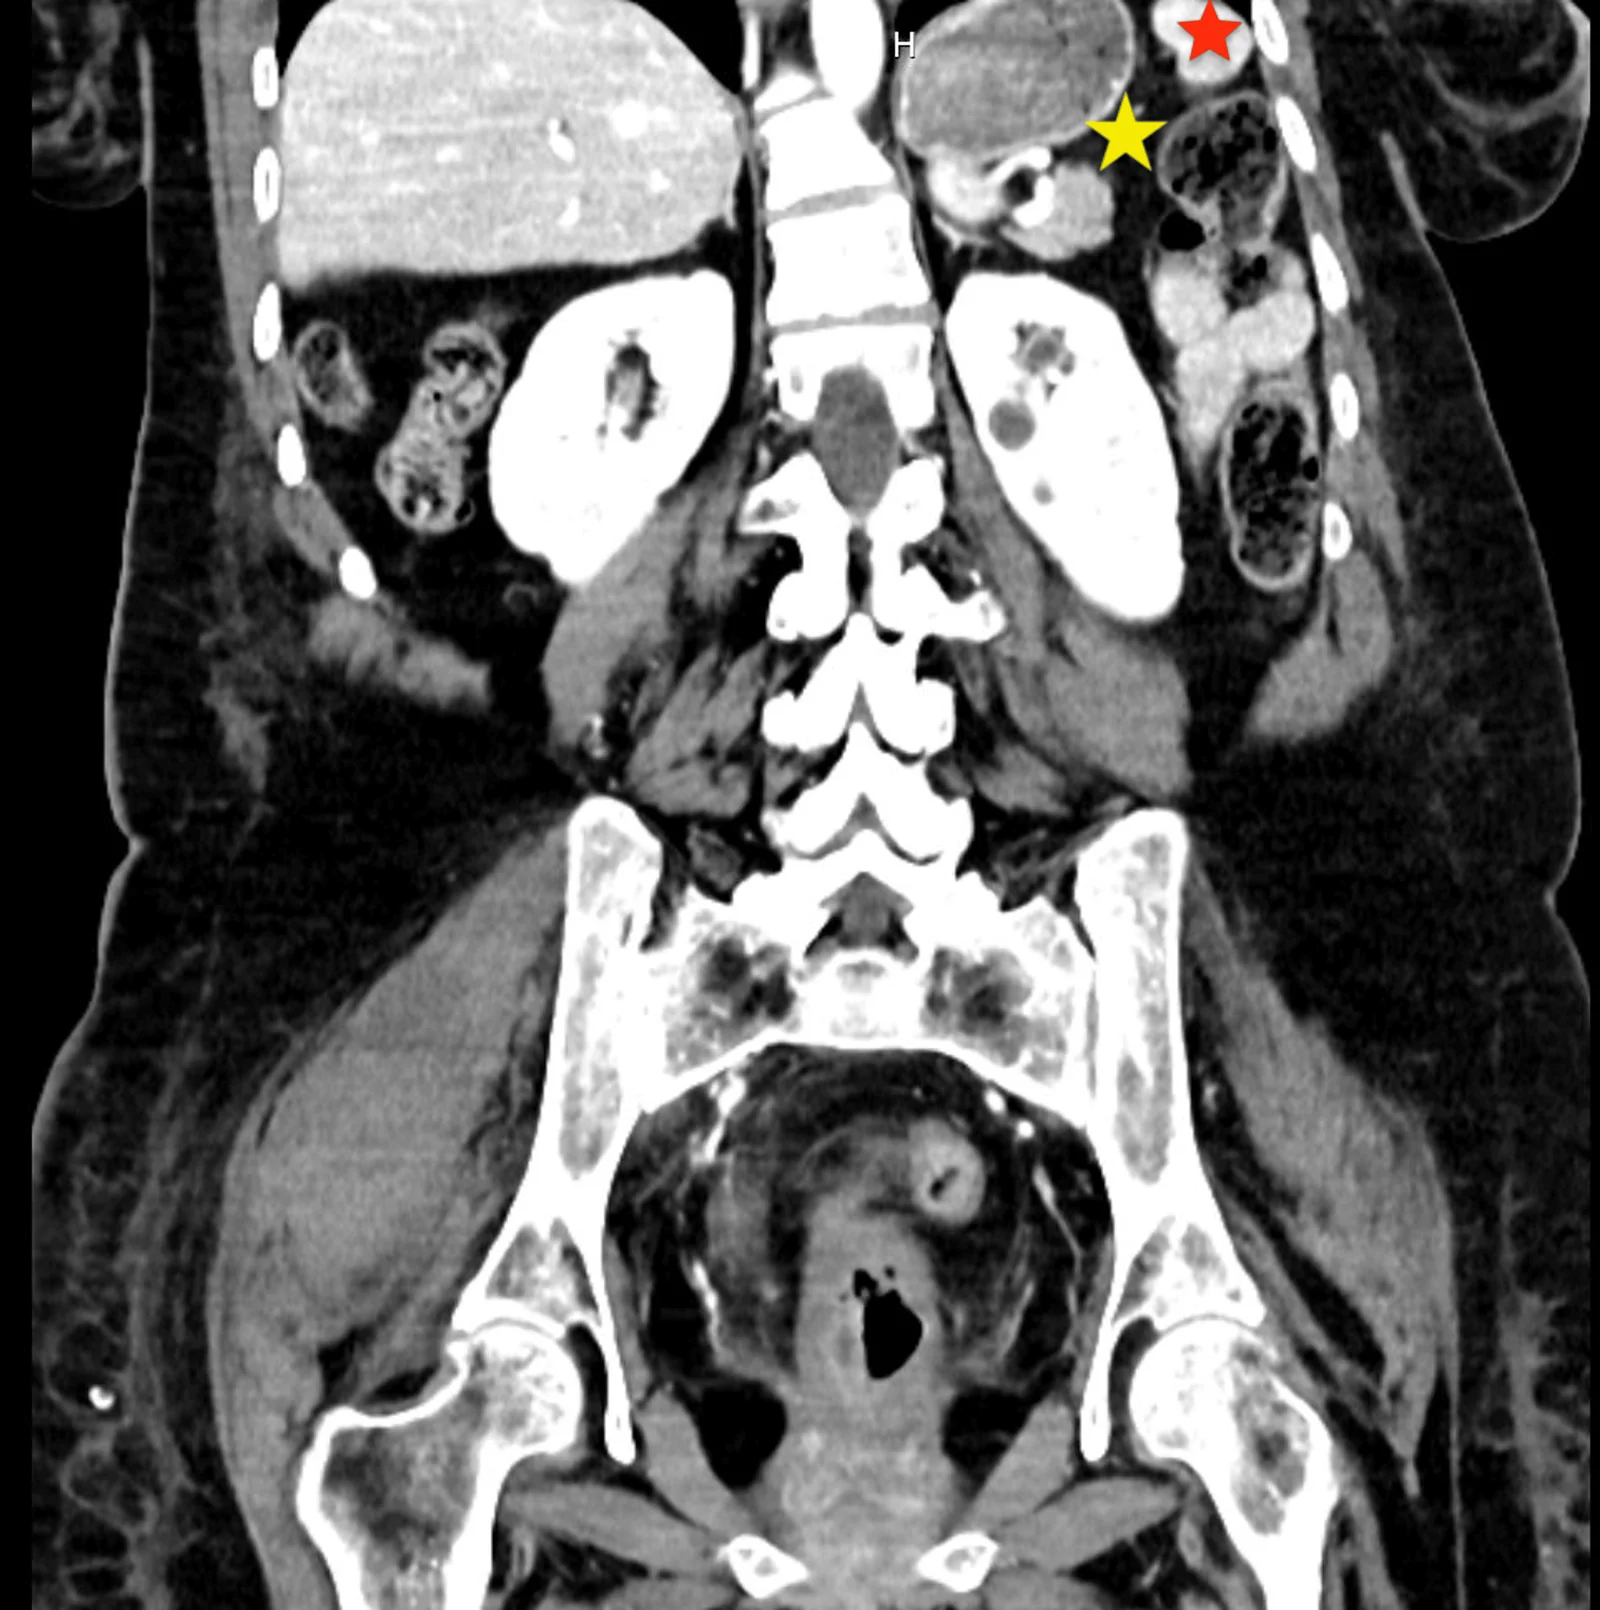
CT abdomen and pelvis with contrast on hospital admission day 1 showing no intra-abdominal hemorrhage or free fluid. The red star indicates the spleen, while the yellow star indicates the retroperitoneal space.
